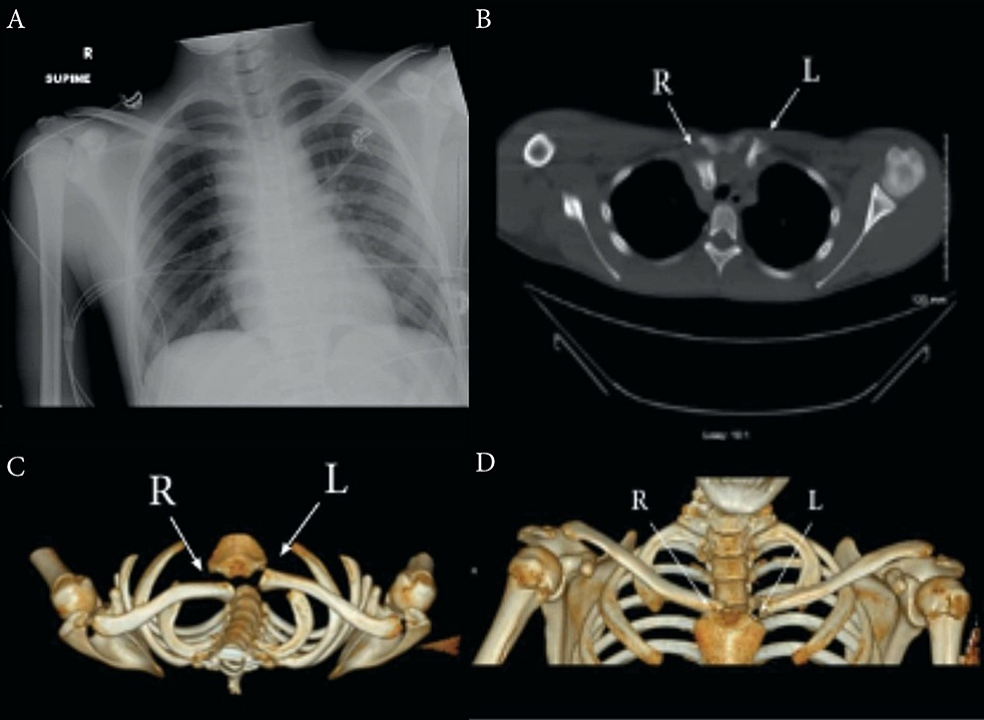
A) Anterior-posterior X-ray of the chest on arrival. B) Axial CT view demonstrating posterior dislocation of the right sternoclavicular joint. C) Axial three-dimensional (3D) CT reconstruction. D) Coronal 3D CT reconstruction. CT: computed tomography, R: right, L: left
